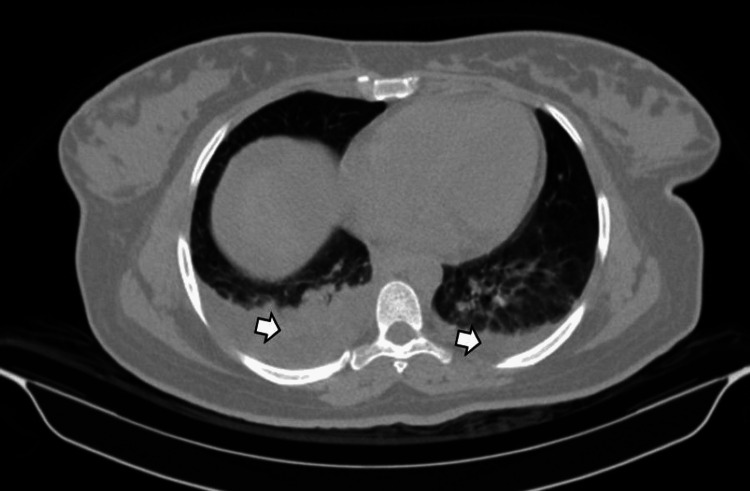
Chest CT image demonstrating bilateral pleural effusions (white arrows) with adjacent passive atelectasis. CT: computed tomography
